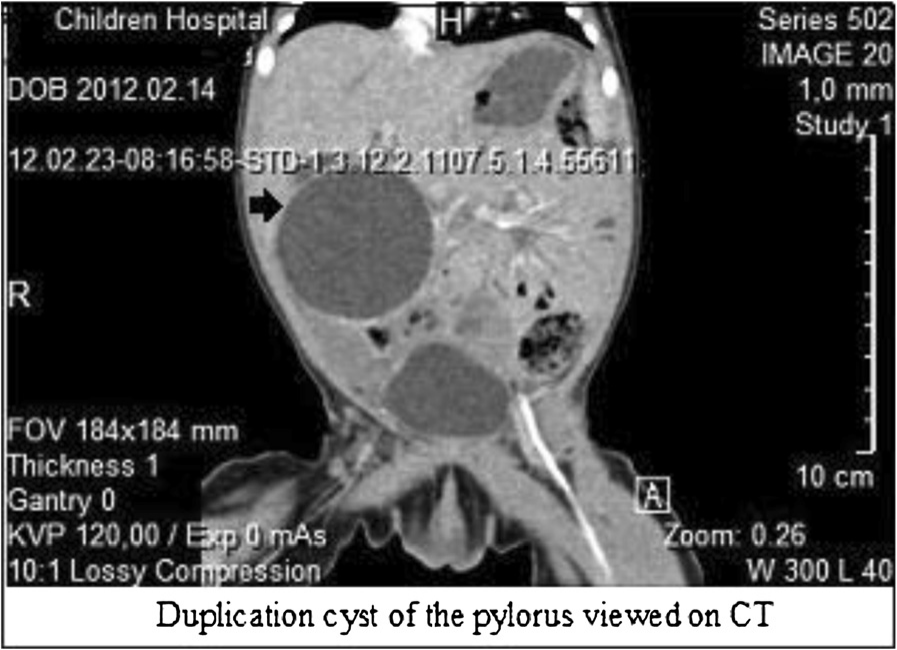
Duplication cyst of the pylorus (arrow) viewed on computed tomography (CT).
